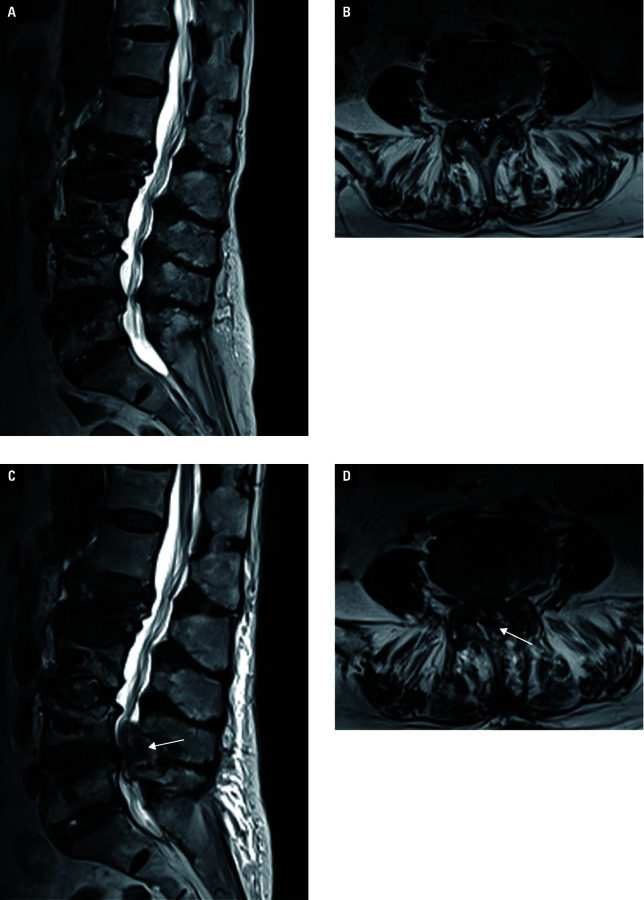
A case of epidural hematoma after unilateral biportal endoscopy (UBE)–unilateral laminectomy bilateral decompression. An elderly woman underwent UBE for lumbar spinal stenosis (A, B). On the second postoperative day, she suddenly developed radiating pain in the right lower extremity with a visual analog score of 9. Magnetic resonance imaging showed formation of an epidural hematoma (C, D; white arrow). The patient did not undergo further hematoma debridement owing to her poor physical condition. After 2 months of conservative treatment, the symptoms were significantly relieved.
